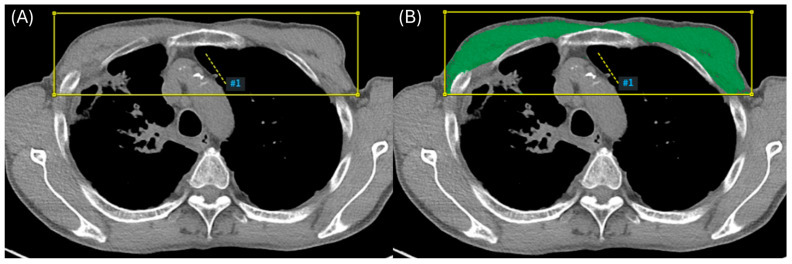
Representative axial CT images at the mid-T4 level from a 72-year-old man, demonstrating pectoralis muscle segmentation using a commercial software program (AVIEW Metrics, Coreline Soft, Seoul, Republic of Korea). (A) Original CT image. (B) Segmented image, with the green area highlighting the segmented pectoralis muscle. The yellow frame and dotted line indicate the selected axial plane at the mid-T4 level, and “#1” denotes the selected slice for analysis.
